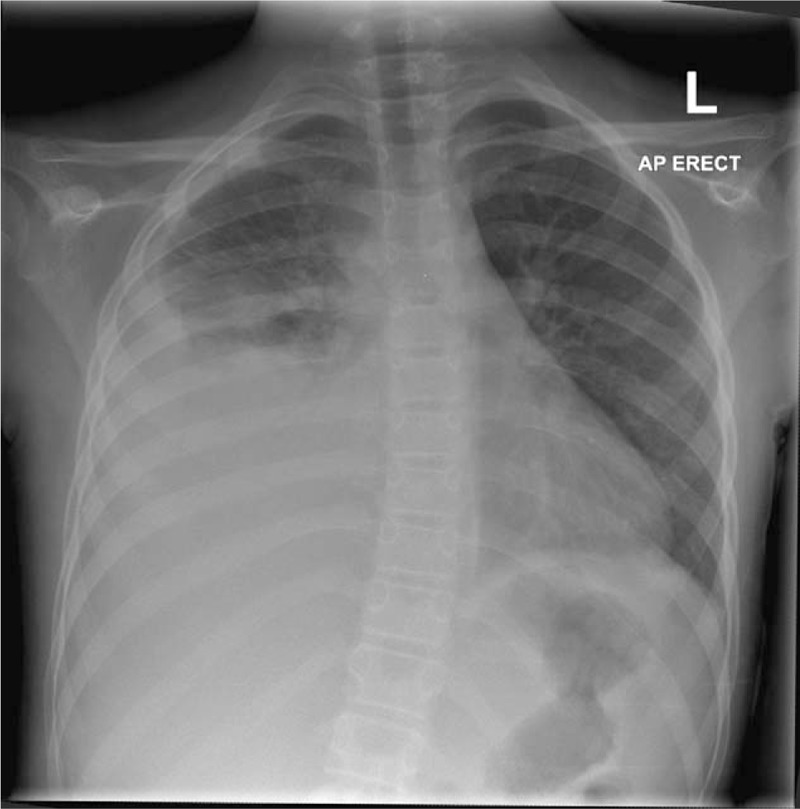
Chest radiograph on day 6 of hospital admission showing a large right-sided pleural effusion.
